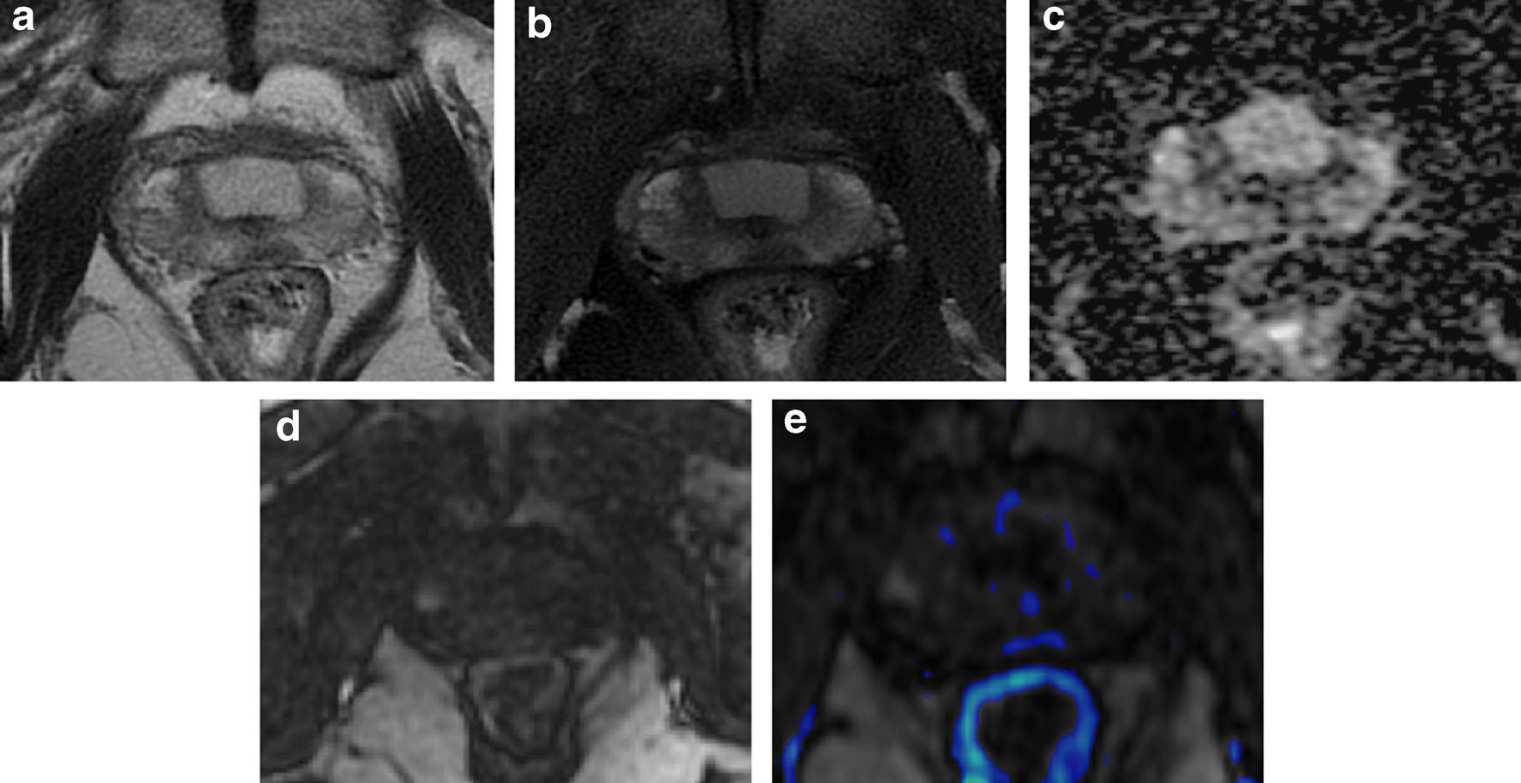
Median posterior hypointense area at the middle third of the gland with no ERC. a Axial T2-weighted fast spin-echo image and b axial Fat Saturated T2-weighted showing an ill-defined hypointense median area at the middle third of the gland. c ADC map showing mild restriction of water molecules diffusivity in the hypointense focus detected on T2-weighted images. d Axial perfusion gradient-echo T1-weighted, and e colour DCE MR map showing mild enhancement of the hypointense zone
